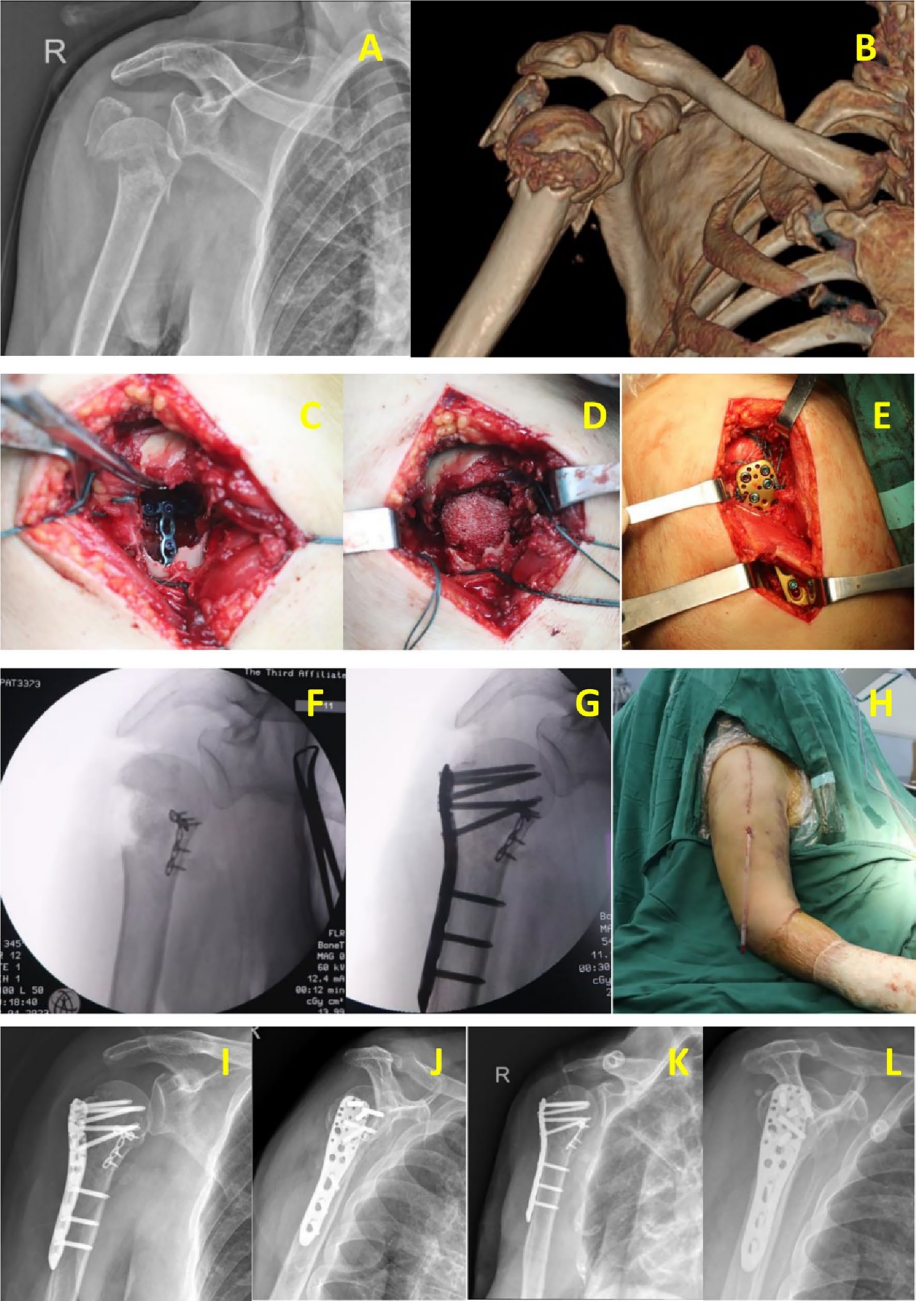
Typical case 1. A 73-year-old woman sustained an unstable proximal humerus fracture (Neer 3-part) from a fall. The pre-operative X-ray and CT scan showed severe displacement of proximal humerus (A, B); The medial supporting plate was used (C); An allogenic allograft was inserted in the bony defect (D); Protection of the axillary nerve (E); Intraoperative X-ray (F, G); Closure of the lateral approach (H). Radiographs 1 month after surgery (I, J); Radiographs 6 months after surgery (K, L)
